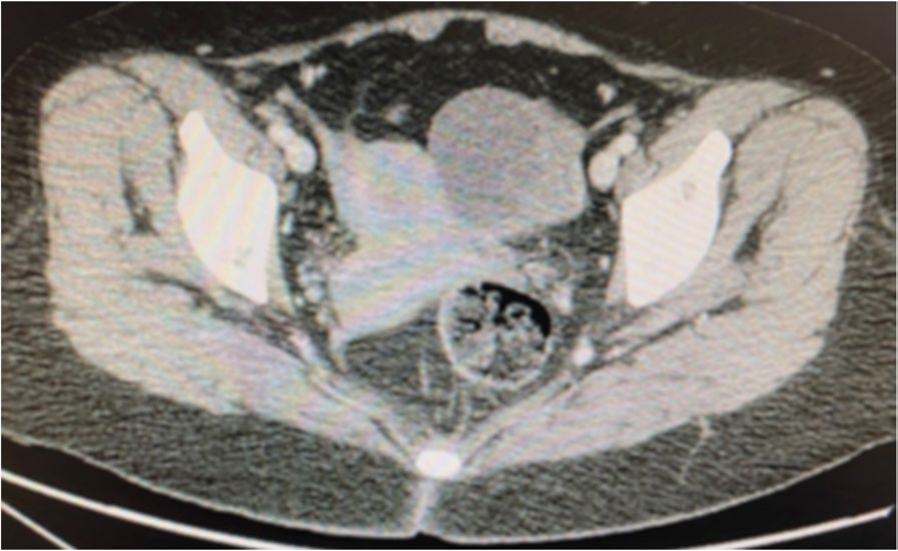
Abdominopelvic CT scan showing the above-described solid-cystic formation measuring 50–60 mm
